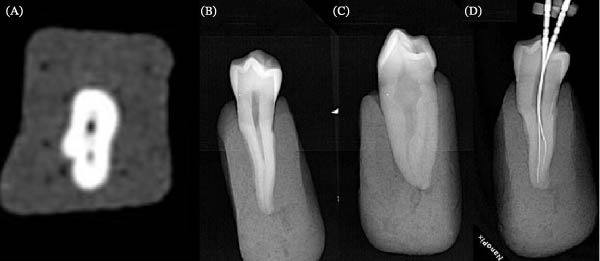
(A) CBCT axial section, (B) buccal view, (C) proximal view, and (D) canal negotiation in a mandibular two‐canal premolar.
